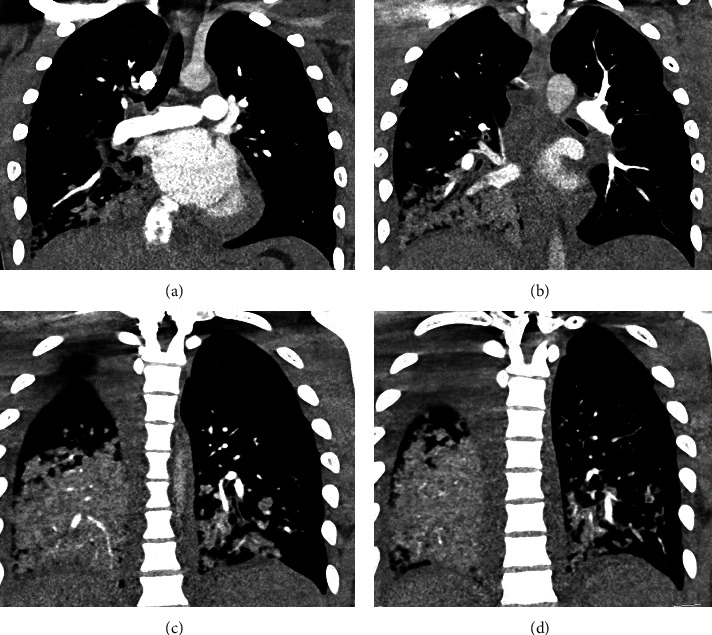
(a–d): Computed tomography angiograms depicting extensive fulminant multifocal bacterial pneumonia with small-moderate bilateral pleural effusions (note the normal appearance of contrast mixing with unopacified inflowing blood in the superior vena cava).
